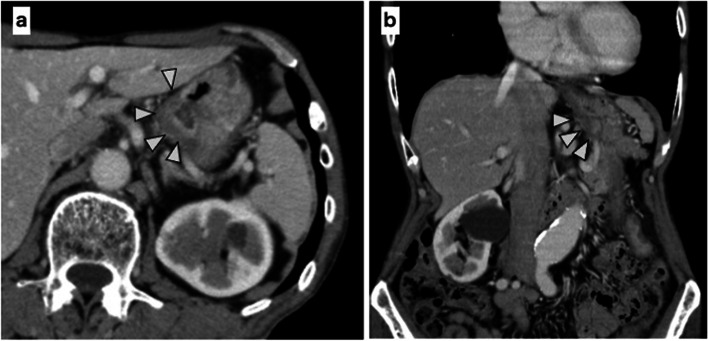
Contrast CT. a, b Contrast irregularity on the side of the lesser curve of the stomach and increased density of fatty tissue
